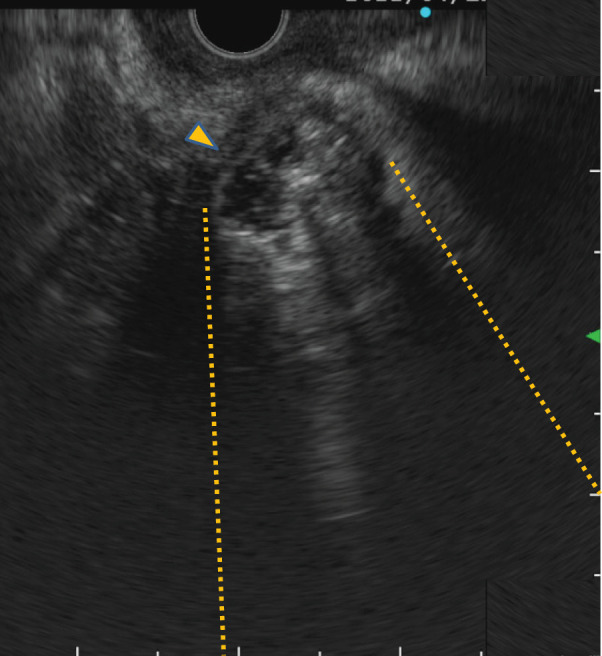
Endoscopic ultrasonography image. The mass has well-defined margins, internal heterogeneity, and a hypoechoic rim at the periphery. Internally, the mass shows areas of high echogenicity with accompanying acoustic shadowing as well as regions of anechoic areas. Arrowhead: mass. Dotted line: acoustic shadowing.
